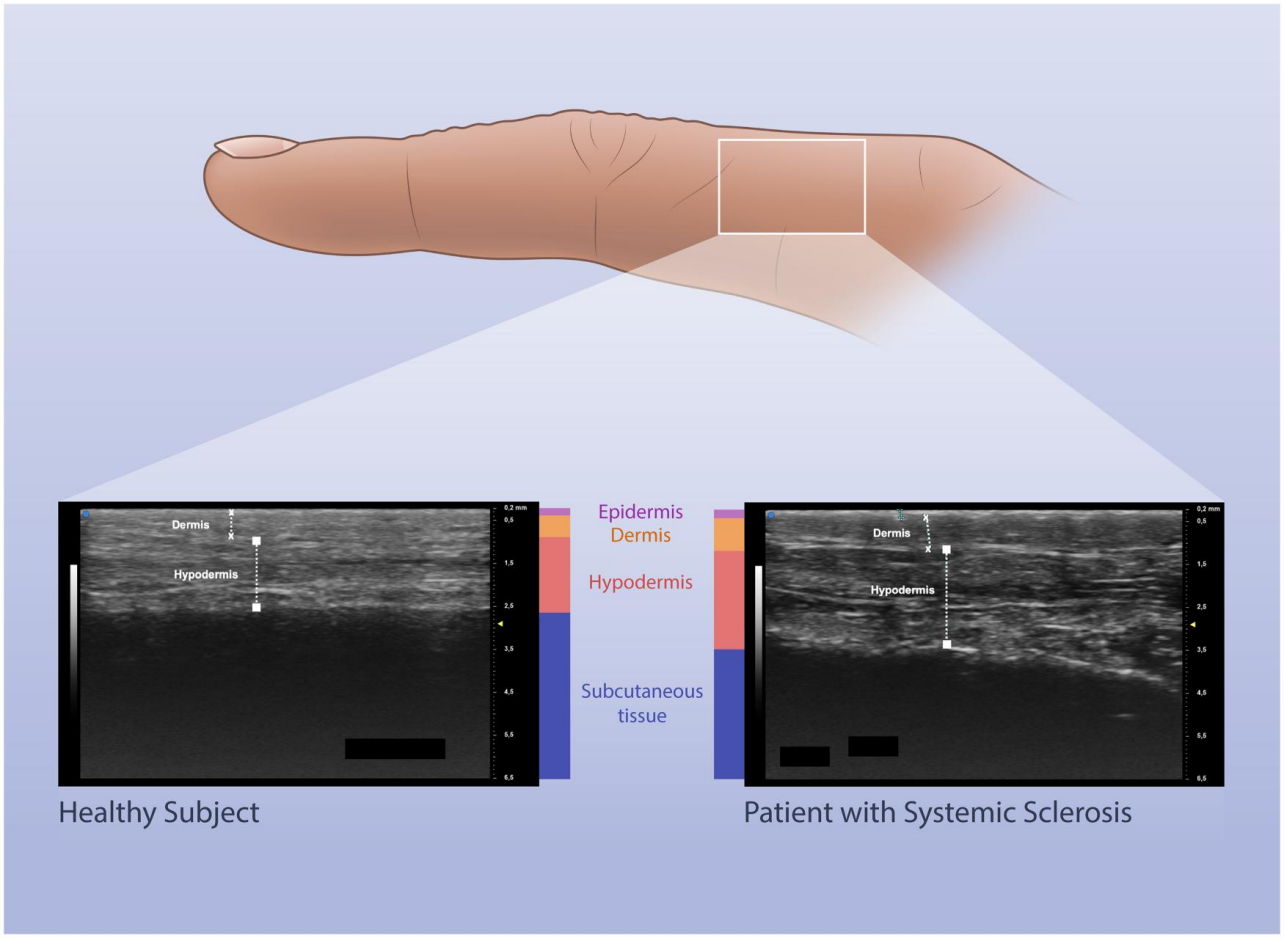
Ultrasonography of skin in SSc. Ultra-high-frequency US (70 MHz) measurement of the dermal and hypodermal thickness of the second finger of the right hand in a healthy subject (left) and in a patient with SSc (right). US images courtesy of Devis Benfaremo
